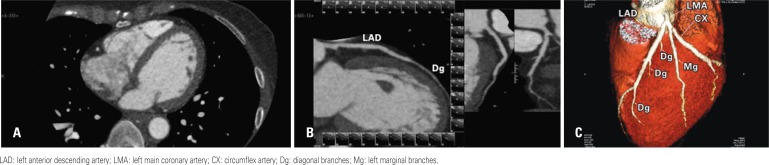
Coronary computed tomographic angiography examination with AIDR-3D. Axial image (A), curved-MIP (B) and 3D volume-rendering (C) reconstructions. Estimated effective radiation dose from coronary computed tomographic angiography only and full examination were 0.43mSv and 1.02mSv, respectively
